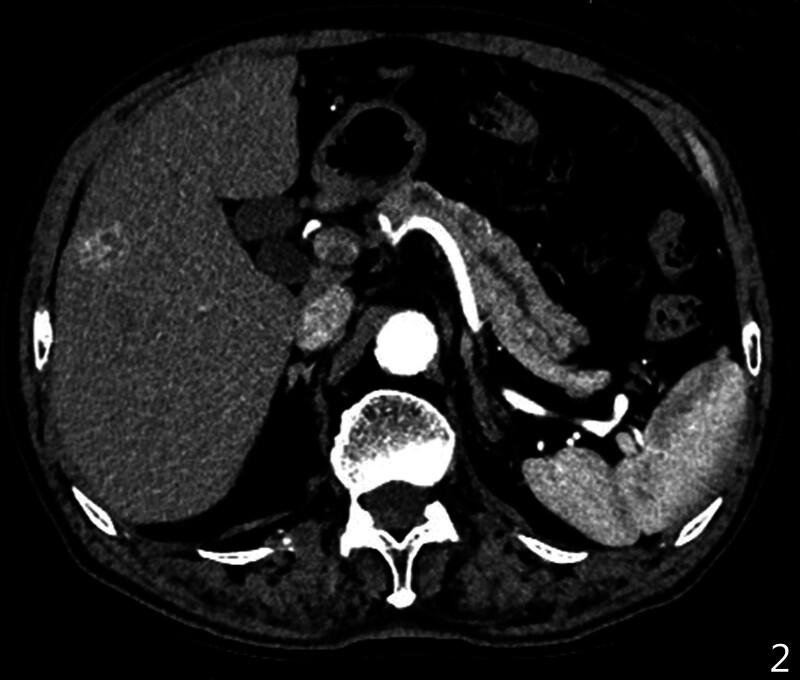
CT shows the dilated pancreatic duct and intrahepatic cholangiocarcinoma located in VIII liver segments. CT = computed tomography.
